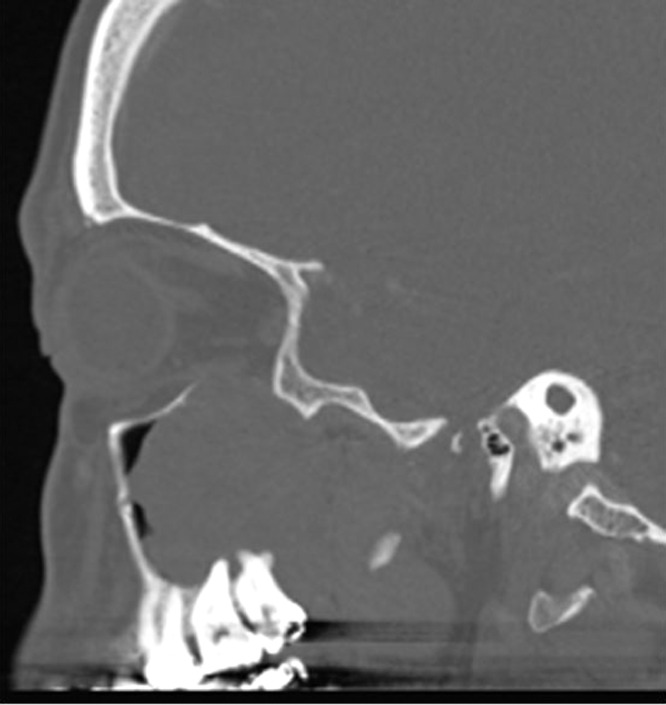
Sagittal CT bone window: The soft tissue mass surrounds the roots of the left second and third maxillary molar teeth without definitive erosion.
